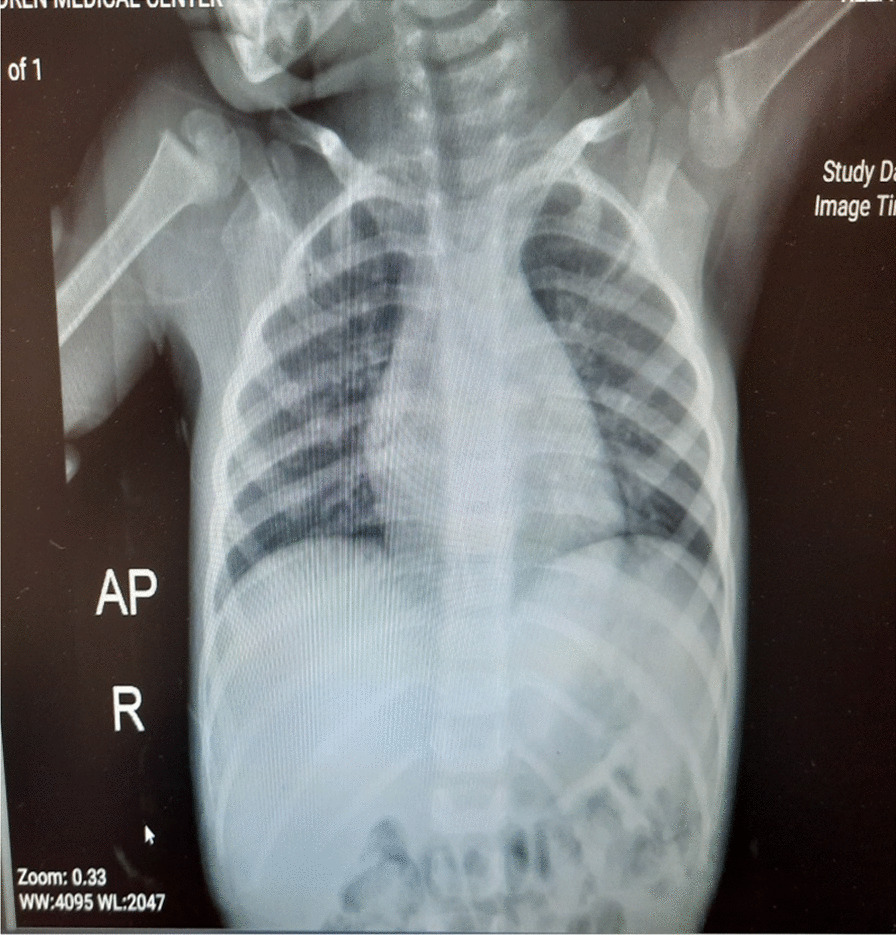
Chest X-ray taken on the second day of hospitalization, showing normal field of both lungs
